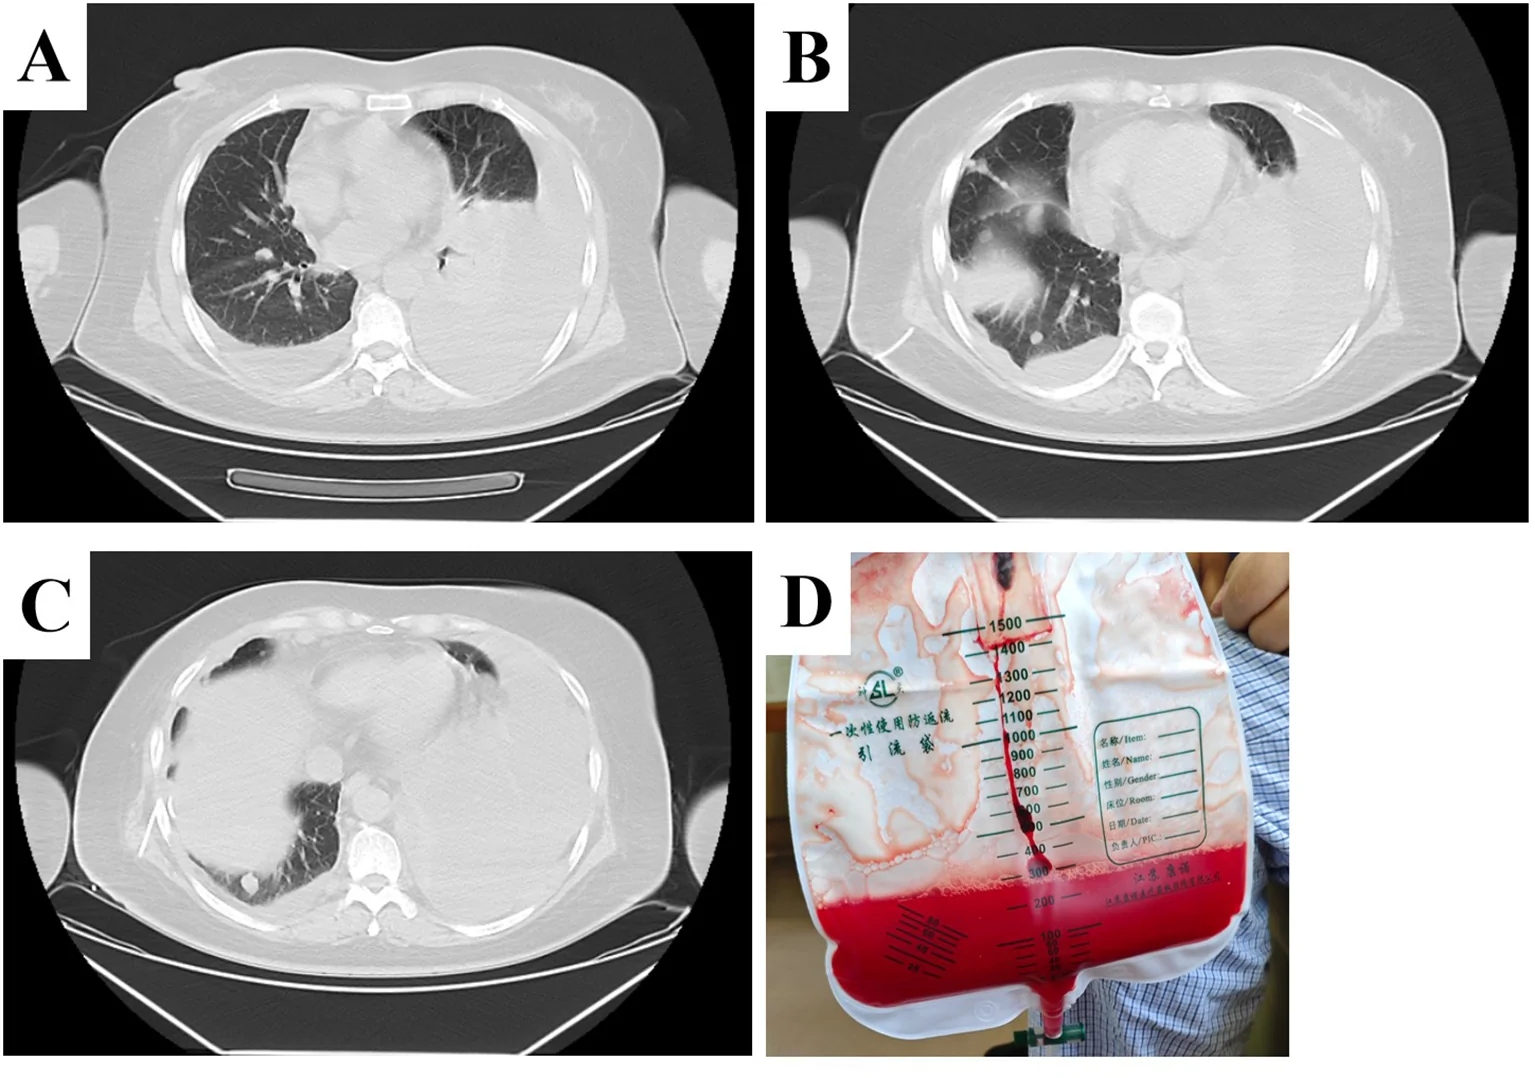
Pre-arterial infusion CT images (April 2026) and drained pleural effusion. (A–C) Chest CT images demonstrating extensive bilateral pulmonary metastatic nodules, pleural thickening, and large right-sided pleural effusion with associated right lower lobe atelectasis prior to regional arterial infusion chemotherapy. (D) Photograph of the hemorrhagic pleural effusion drained from the right thoracic cavity, consistent with malignant etiology.
